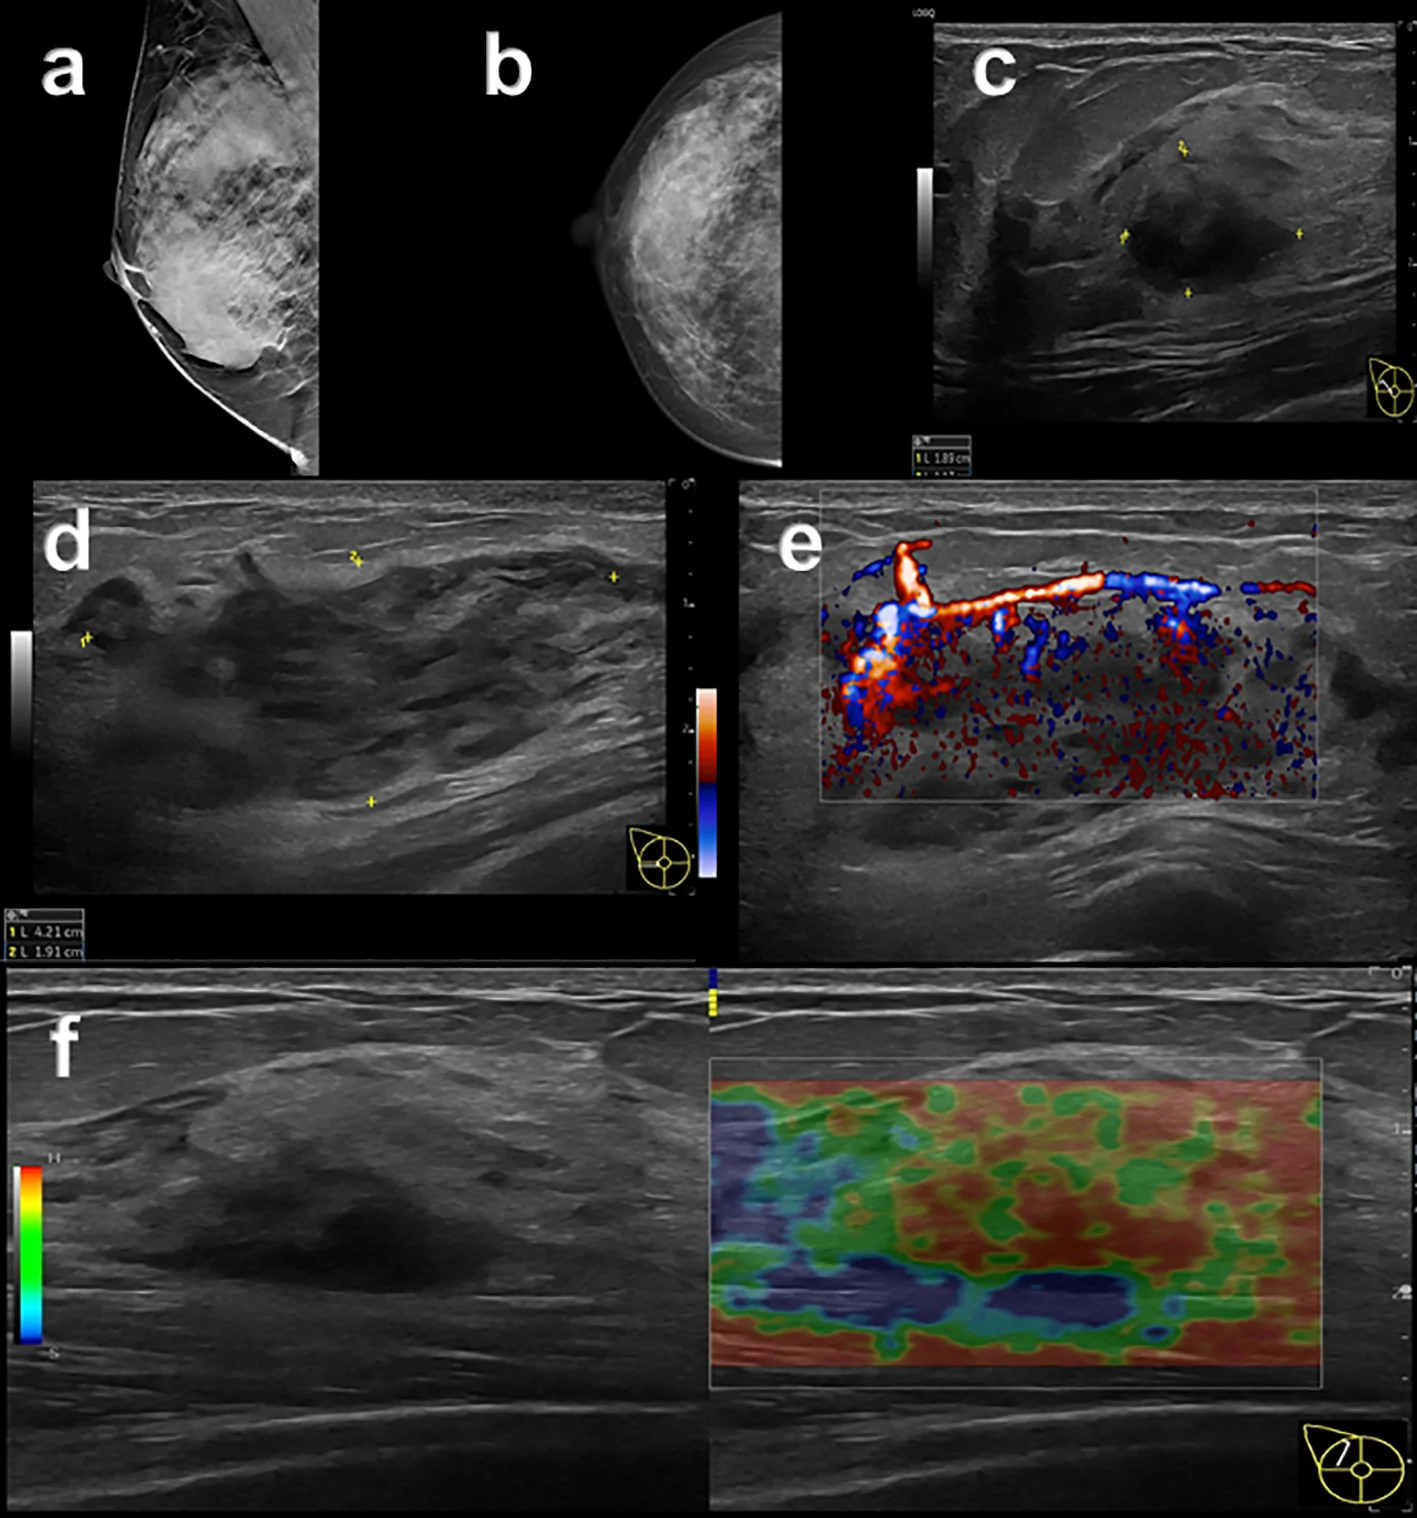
Preoperative imaging findings of the right breast. (a) Digital breast tomosynthesis (mediolateral oblique slice) and (b) full-field digital mammography (craniocaudal 2D view), showing a periareolar focal asymmetry with indistinct margins within a heterogeneously dense right breast (ACR category C). (c) Ultrasonography at the 10 o’clock position: an oval to lobulated mass with obscured margins and heterogeneous echogenicity. (d) Ultrasonography at the 9 o’clock position: a lobulated hypoechoic mass with indistinct margins and a peripheral echogenic rind. (e) Color Doppler flow imaging (CDFI) demonstrating predominantly peripheral vascularity in the 9 o’clock lesion. (f) Elastography showing intermediate-to-hard tissue stiffness of the 9 o’clock lesion (H, hard; S, soft). Both lesions were classified as BI-RADS category 4A.
